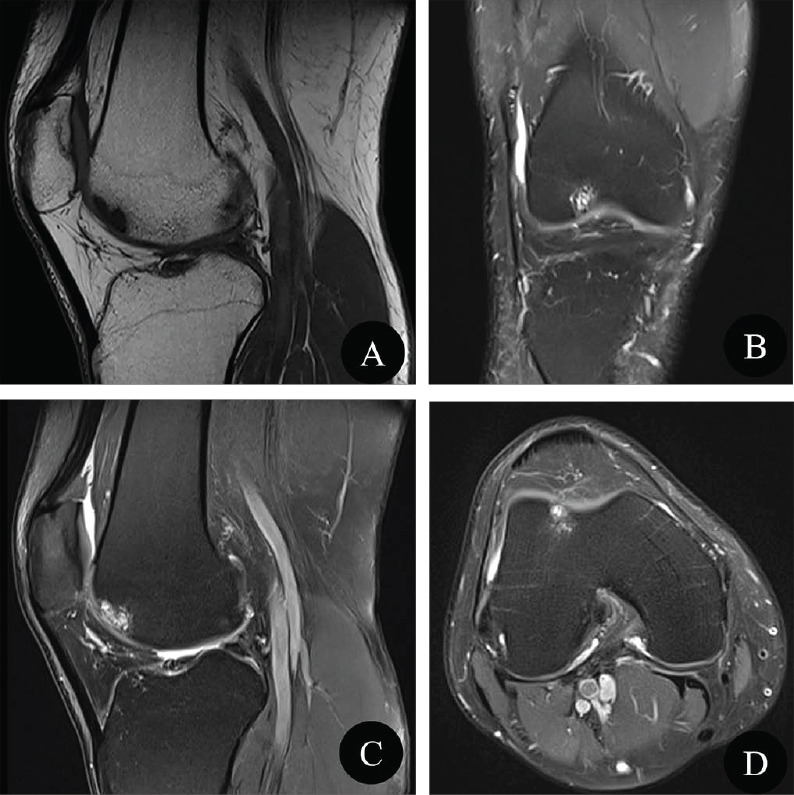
Initial presentation of the right knee MRI examination. Sagittal T1-weighted image (A, T1WI), coronal fat-suppressed proton density-weighted image (B, PDWI-fs), sagittal PDWI-fs (C), and axial PDWI-fs (D) showed the unstable OCD lesion of the lateral femoral condyle. MRI images showed that the local articular cartilage of the lateral femoral condyle was thinner and heterogeneous, had slightly higher signal intensity on the PDWI-fs image, and was surrounded by multiple small cysts in the subchondral bone.
